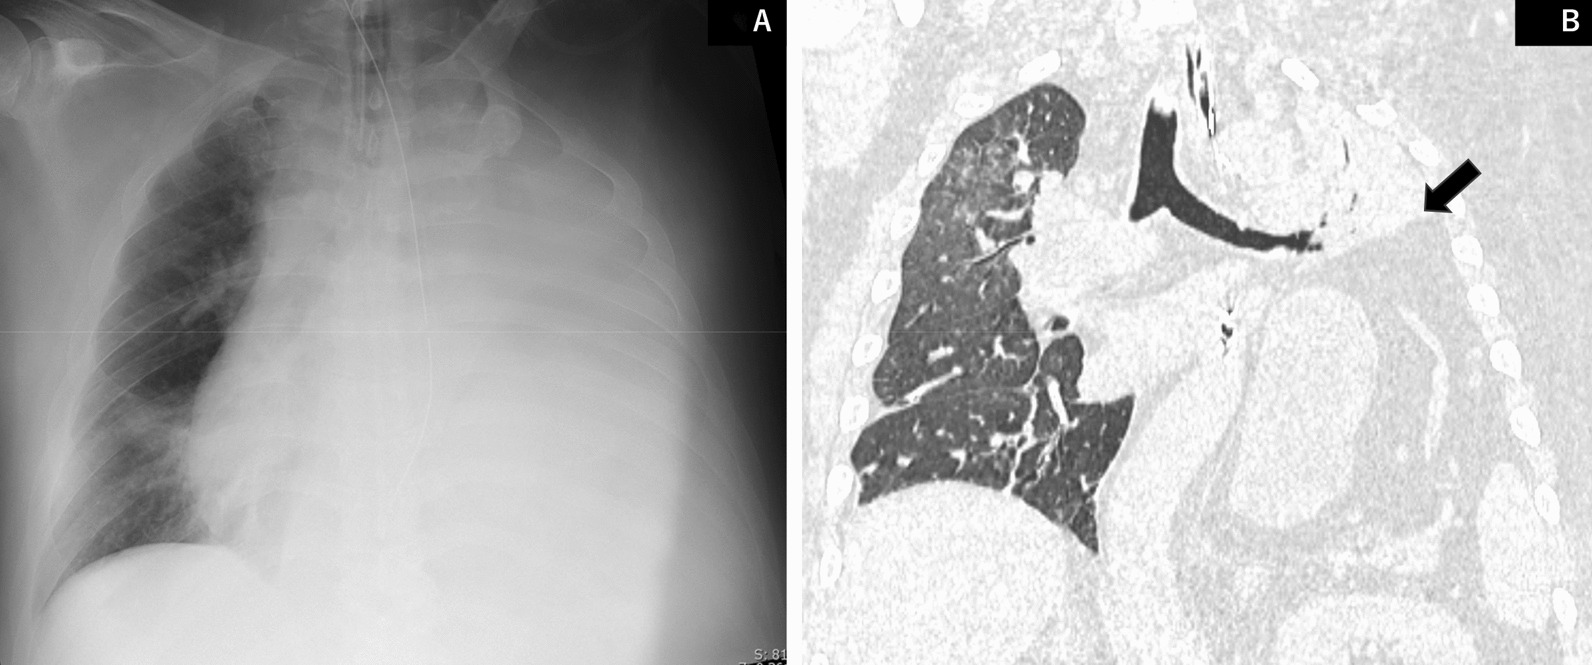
A Chest X-ray findings on admission showed loss of air content in the left lung. B Coronal computed tomography findings on admission showed slight ground-glass areas in the upper lobe of the right lung. The left lung showed almost complete loss of air content and elevated left diaphragm, which is indicated by a black arrow
